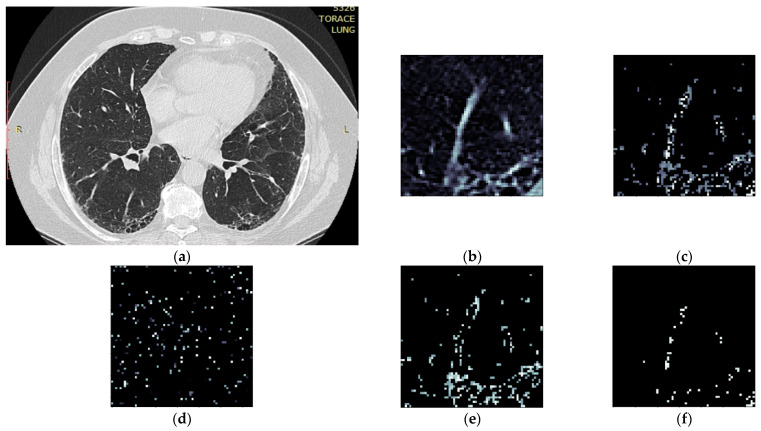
Splitting CT sample into layers (a) original CT, (b) sample crop, (c) combined Emphysema, GGO, and Consolidation layers, (d) Emphysema layer, (e) GGO layer, (f) Consolidation layer.
